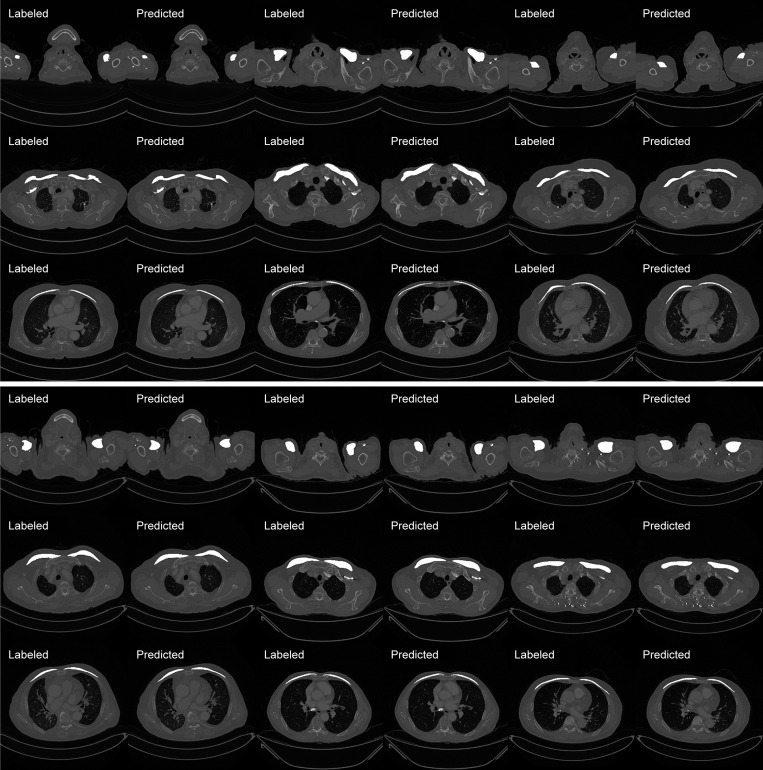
The labeled image of the patient CT dataset and its predicted result using the proposed method.
